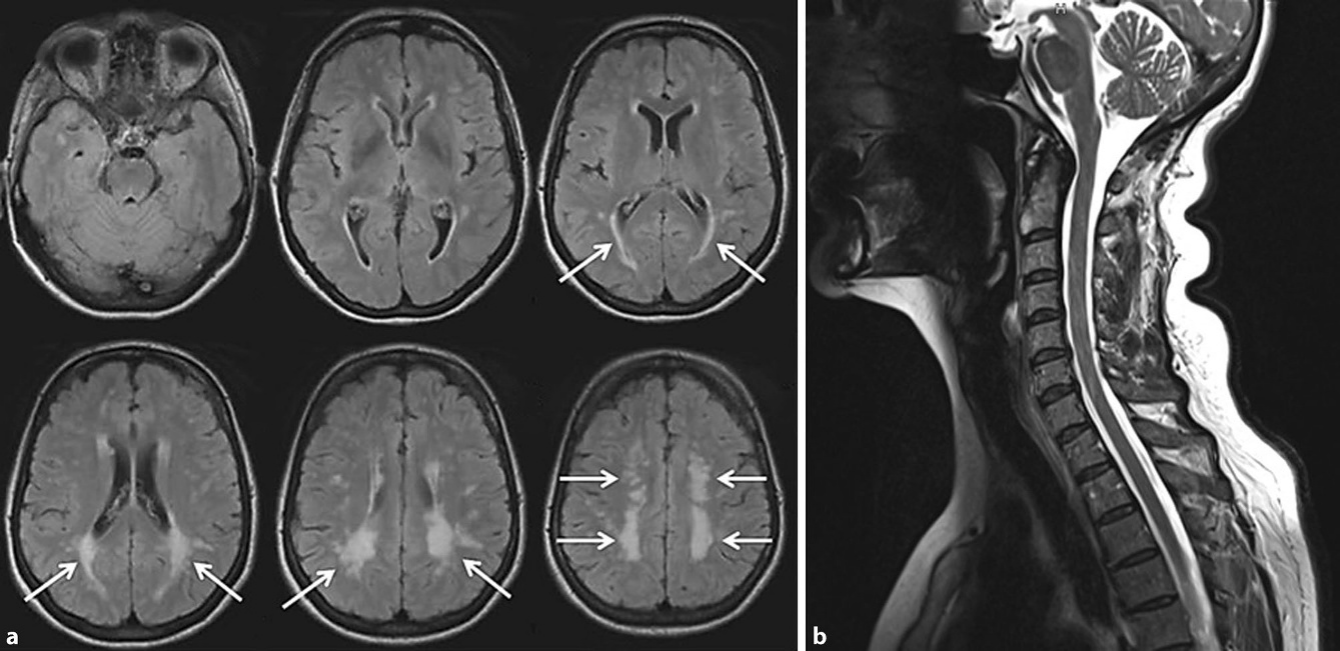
Magnetic resonance images of the brain (a) and spinal cord (b). a shows six axial FLAIR sections through the brain in craniocaudal direction starting at the brainstem. Extensive and rather symmetric white matter changes (*arrows*) located preferentially in both parietal lobes and along the lateral ventricles are seen. The subcortical U-fibres and infratentorial brain parenchyma are spared. b is a sagittal-T2-weighted section of the cervical and upper thoracic spine. There are some artifacts but no definite lesion is seen in the spinal cord. *FLAIR* fluid-attenuated inversion recovery
